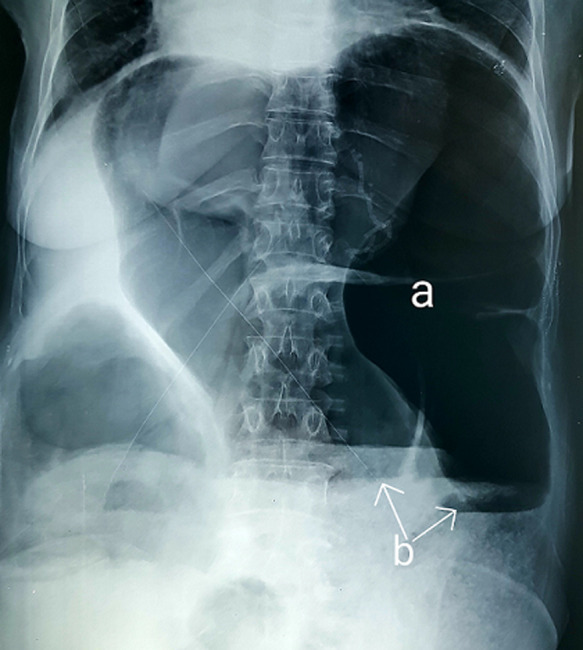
plain abdominal X-ray showing dilated colon (a) and air-fluid levels (b)
